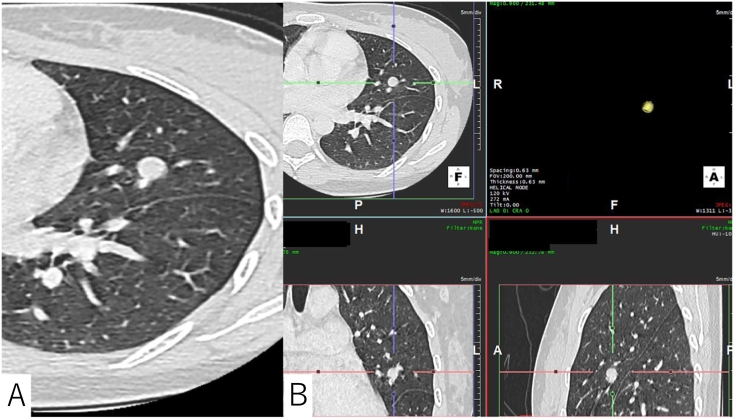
A: A chest CT showing a 9 mm pulmonary nodule with a round shape and smooth margin in the left upper lobe. B: The volume of the nodule was estimated to be 425 mm3 based on the three-dimensional CT volume rendering reconstruction.
